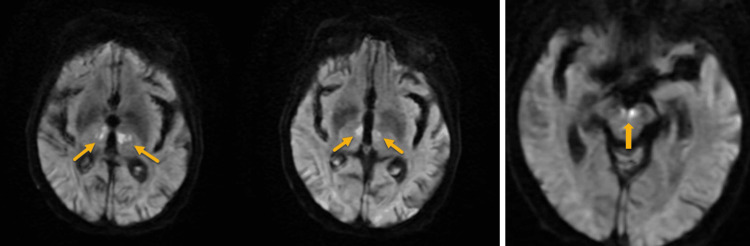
Diffusion-weighted sequence of MRI brain showing symmetrical diffusion restriction in the paramedian aspect of bilateral thalami and midbrain region, suggesting an acute infarction (as indicated by arrows).
